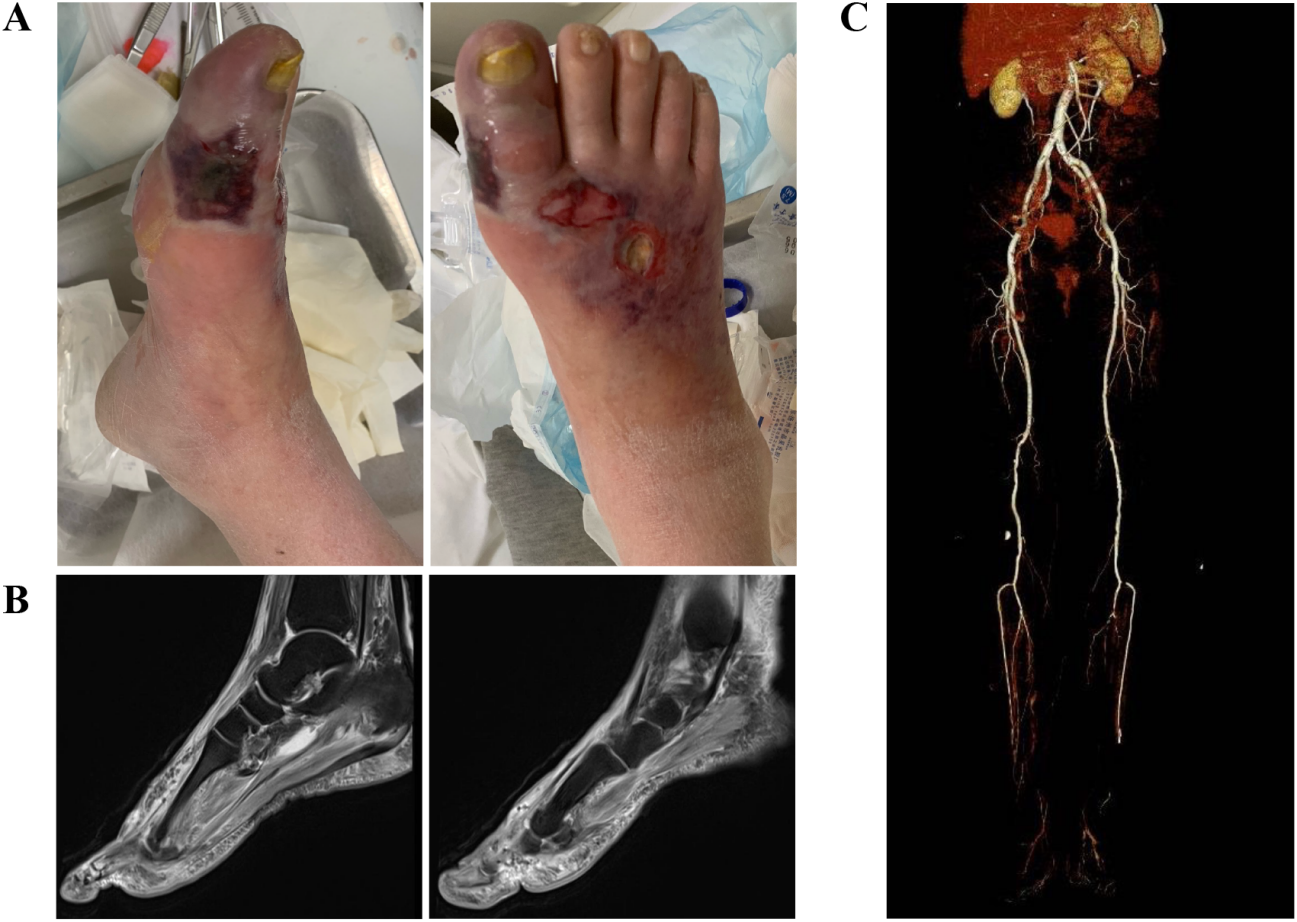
Initial presentation showing a diabetic foot ulcer involving deep tissues and ischemic changes. (A) Foot wounds;(B) Magnetic resonance imaging (MRI) of the foot; (C) Four-dimensional computed tomography angiography (4D-CTA) of the lower extremities;.
